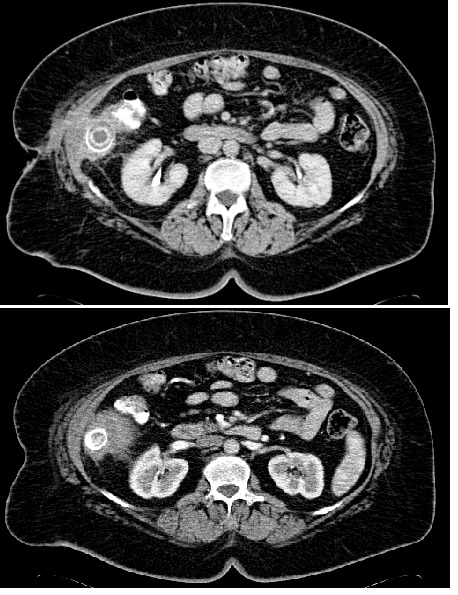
Abdominal CT upon admission- a central, round, partially calcified structure of about 3.2 cm; adjacent to this, there is a fistulous tract towards the skin
